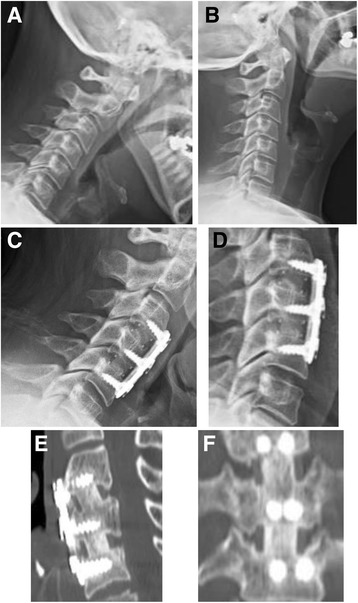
Two-level ACDF using Trinity Evolution that was performed on a 44-year-old obese female at C3-4 and C4-5. a Pre-operative flexion radiograph. b Pre-operative extension radiograph. c Twelve month flexion radiograph. d Twelve month extension radiograph. e Twelve month sagittal CT. f Twelve month coronal CT
